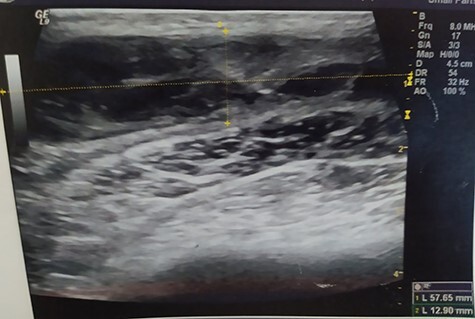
Pelvic ultrasound indicating an acute/nonorganized hematoma into the fatty tissue of the right iliac fossa, with thrombotic features.
